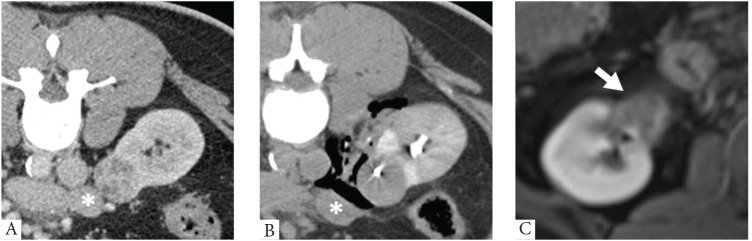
Ancillary protective maneuver using carbo-dissection in low-risk mRENAL score. (A) Intra-procedural contrast-enhanced CT showing an anterior exophytic right renal mass in contact with small bowel loops (white asterisk). (B) Post-treatment showing carbo-dissection displacing the right peri-renal structures. A radio-dense intra-tumoral coil marker inserted before treatment is also seen (C) 1-year post treatment follow-up of MRI showed no enhancement at the site of cryoablation (white arrow), suggesting the absence of disease recurrence.
